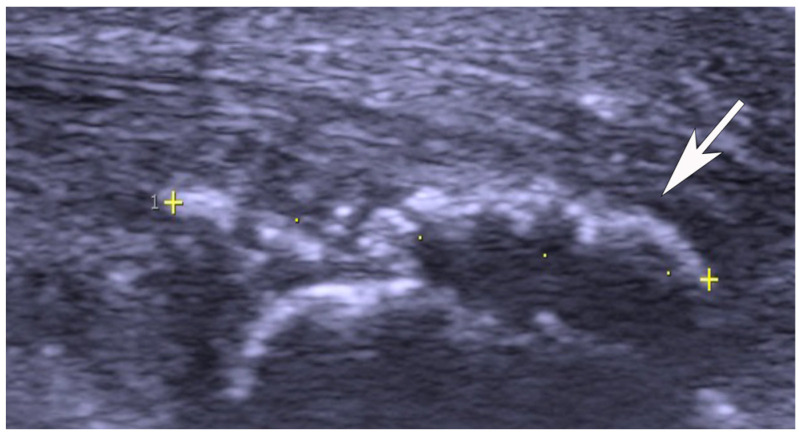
Ultrasound and colour Doppler image showing calcifications and bone formations inside the tendinopathy of the Achilles insertion (arrow and cross).
